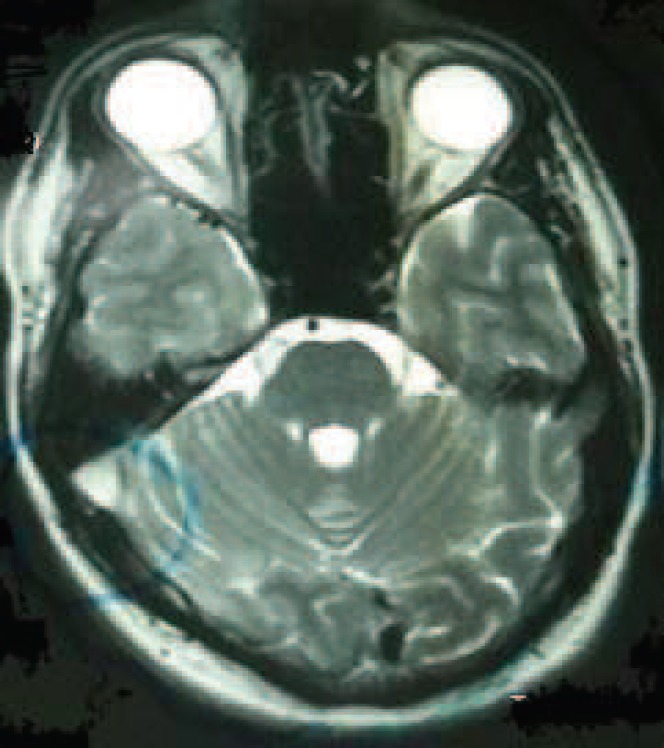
Axial brain MRI in T2-weighted sequence showing abnormal signals in sigmoid sinus.
